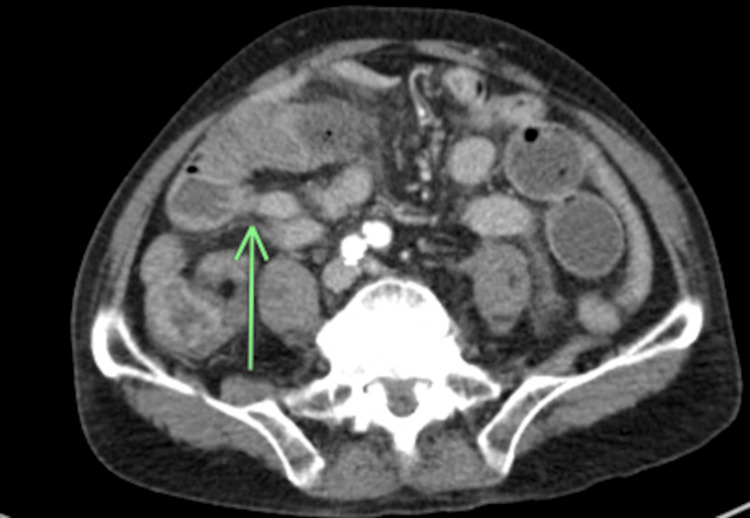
Axial CT of the abdomen and pelvis demonstrating moderate- to high-grade small bowel obstruction There is fecalization to the level of a transition point (green arrow) in the distal small bowel in the right lower quadrant. There is diffuse distension with dilated segments of the small bowel. No pneumatosis or free air was seen.
